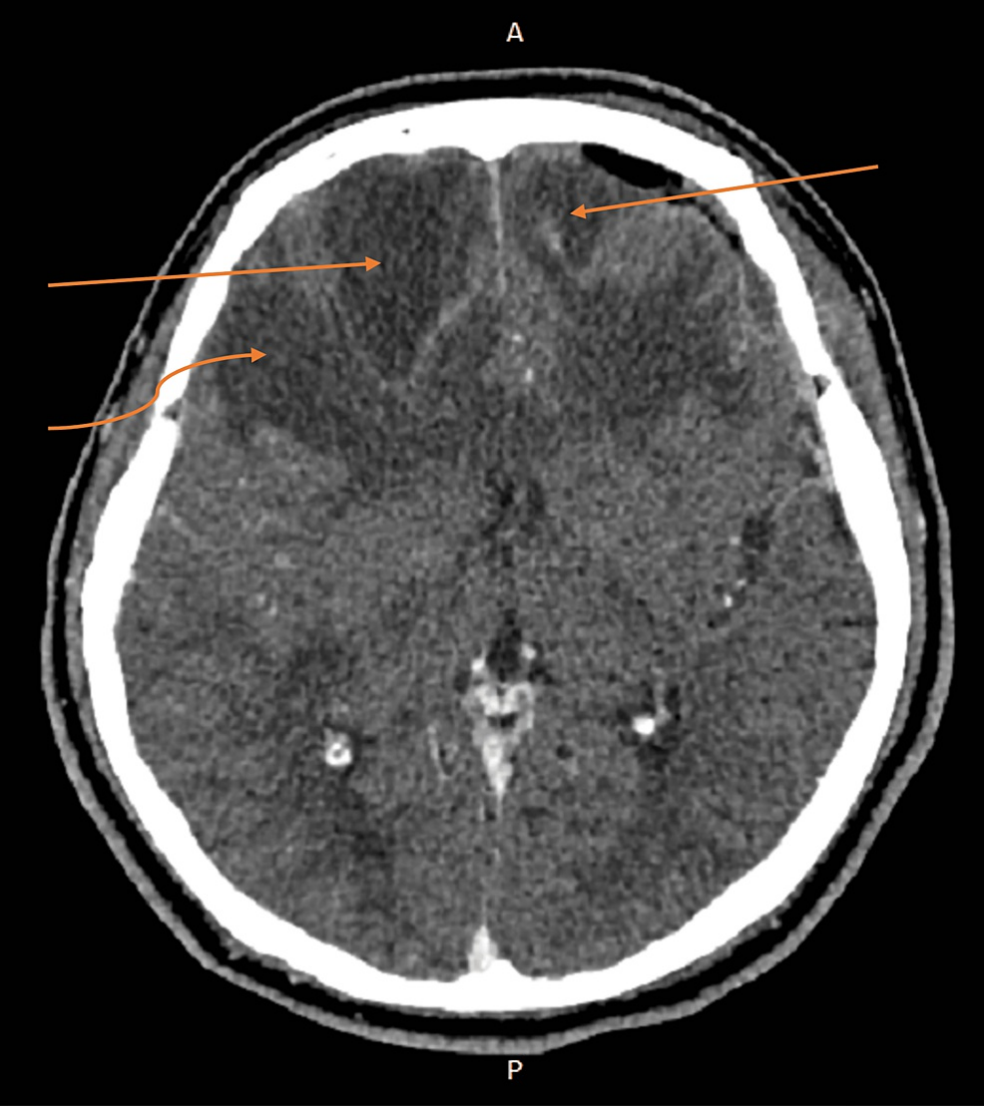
Repeat CECT six weeks after systemic antibiotic therapy shows ill-defined hypodense lesions within the bilateral frontal region with the right frontal lesion measuring 3.9 cm x 3.2 cm and the left frontal lesion measuring 2.0 cm x 2.2 cm (straight arrows). Also seen is an ill-defined hypodense lesion within the right temporal lobe measuring 2.8 cm x 2.4 cm (curved arrow). CECT: Contrast-enhanced computed tomography
